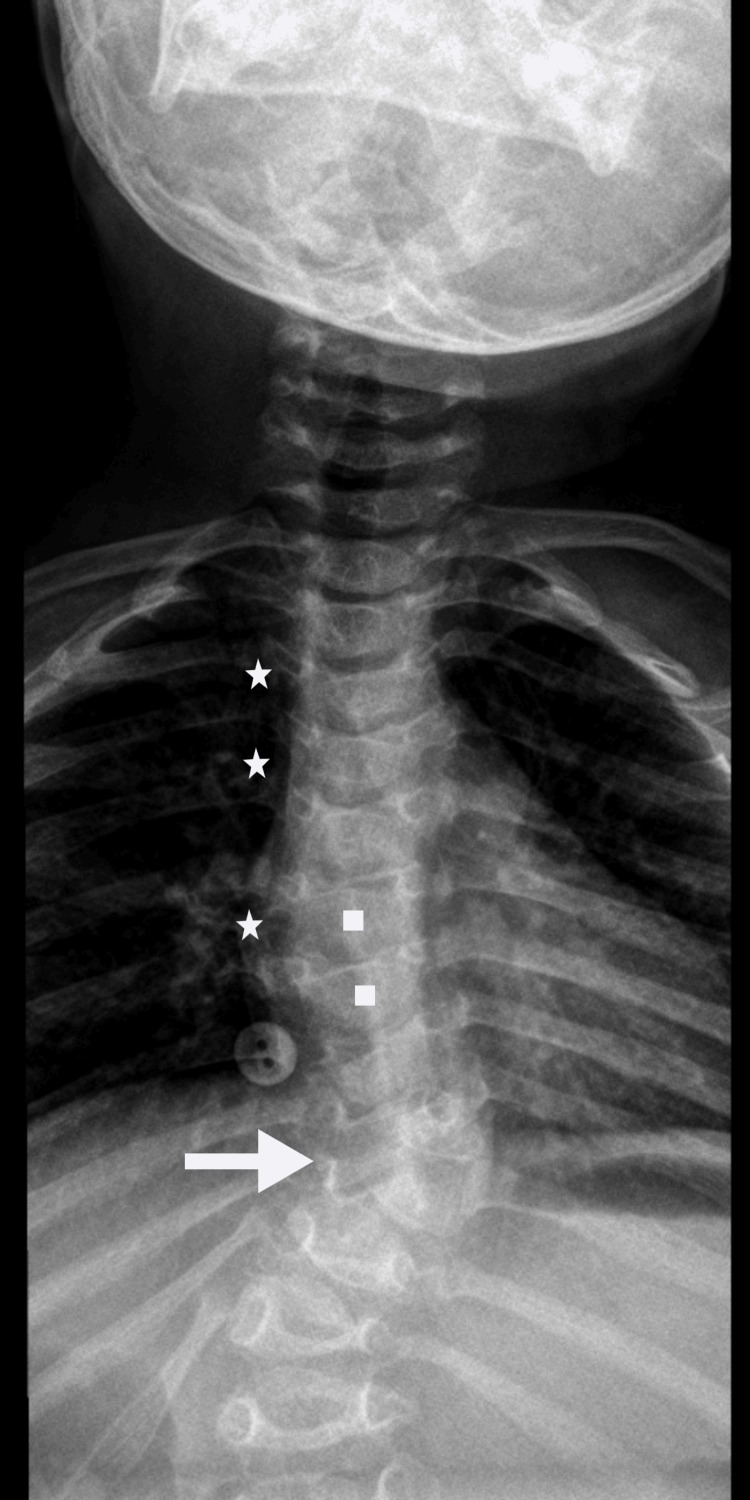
Spine radiography of the patient. The image shows calcifications of the epiphyseal cartilages in the anterior costal arches and the vertebral column (stars), scoliosis (arrow), and vertebral dysmorphisms, such as reduced height of vertebral bodies and rounded morphology of vertebral bodies at the dorsolumbar level (squares).
